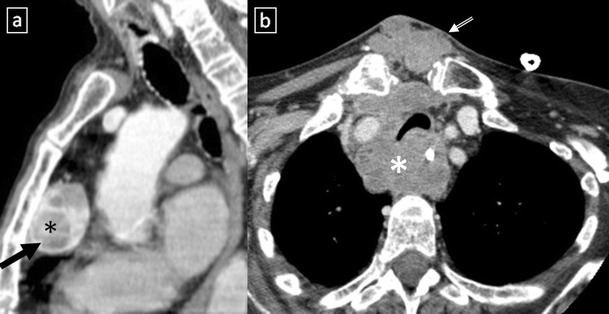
a Heterotopic mediastinal goitre. Sagittal contrast-enhanced CT scan demonstrates a well-defined mass (*arrow*) located in the retrosternal space which shows an intense and heterogeneous contrast enhancement due to the presence of cystic areas (*). No connection to the thyroid gland from the neck. b Anaplastic thyroid carcinoma in a 64-year-old woman with respiratory failure. Contrast-enhanced CT scan shows an anterior mediastinal soft tissue mass (*) which surrounds great vessels and oesophagus and compresses trachea. Note the extension to the suprasternal fossa (*open arrow*)
